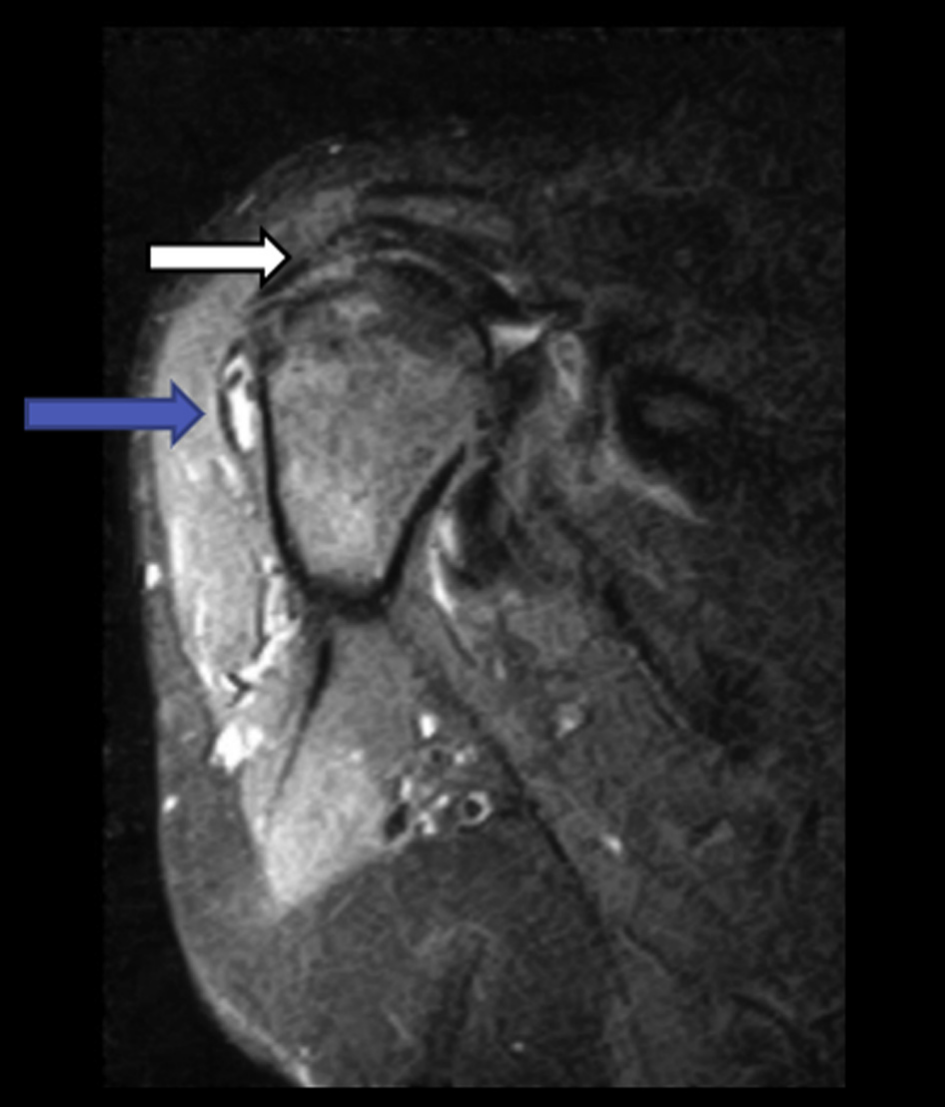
T2 inversion recovery coronal image of partially intact graft. The image displays a postoperative MRI of a patient after superior capsular reconstruction that is partially intact. Note the heterogenous pattern of the graft at white arrow. This finding likely represents graft breakdown, resorption, or only partial vascularization of the graft. Note the increased fluid about the graft in the subacromial space at blue arrow. (MRI, magnetic resonance imaging.)
